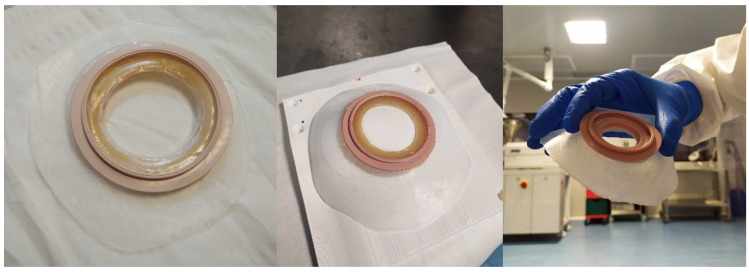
The three stoma patches produced for a patient of the clinical trial. From left to right, using CT scan, Structure Sensor and Go!Scan 20.
